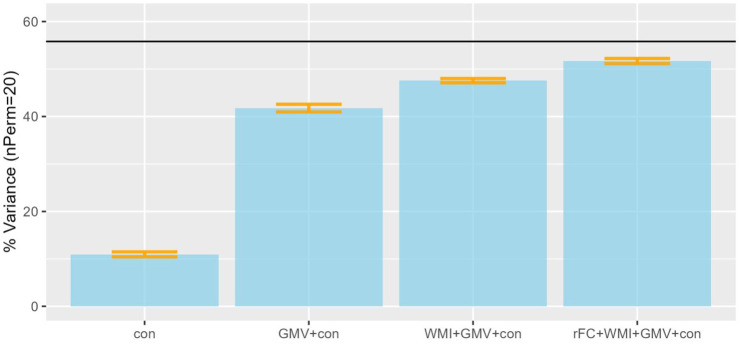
The proportion of variance in fluid intelligence explained by various brain metrics of N = 586 adults aged from 18 to 88 in the Cam-CAN Stage 2 data (www.cam-can.org). The horizontal line is an estimate of the ‘noise-ceiling’, that is, maximum explainable variance based on reliability of the Cattell test of fluid intelligence (estimated from an independent cohort). Variance explained was estimated using 10-fold, nested cross-validation of a linear model with L1- and L2-regularisation, with error bars from 20 permutations. The left bar reflects potential confounds (con), such as sex and polygenic score for intelligence. The next three bars reflect variance explained after stacking predictions from additional: (1) grey-matter volume (GMV) from 98 ROIs in the Harvard-Oxford atlas after segmenting a T1-weighted MRI; (2) white-matter integrity (WMI) from 48 ROIs in the Julich atlas using mean signal kurtosis from a Diffusion-weighted MRI; (3) resting-state connectivity (rFC) from 153 connections within and between 17 Yeo networks from BOLD-weighted MRI. Of course, it is possible that multiple brain properties interact in determining cognition, which could be modelled by adding multiplicative relationships. Data and code are available from https://github.com/RikHenson/CogRes
